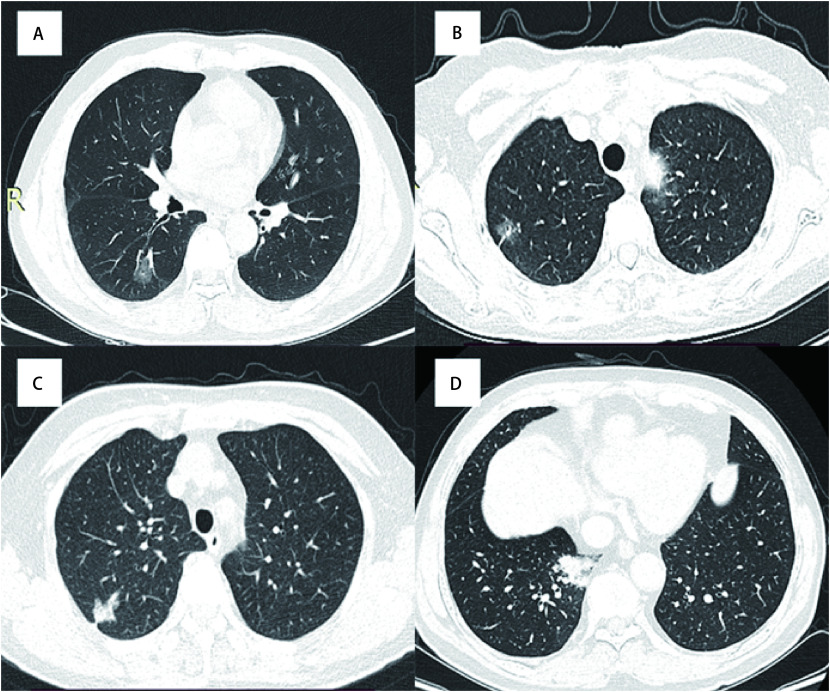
典型的CT征象。A：*EGFR*、*ALK*基因野生型腺癌，以磨玻璃成分为主；B：*EGFR*基因外显子21突变型腺癌，存在胸膜牵拉征及较多磨玻璃成分；C：*EGFR*基因外显子19突变型腺癌，存在分叶征及较多实性成分；D：*ALK*基因突变型腺癌，以实性肿块为主。 Typical CT signs. A: *EGFR* and *ALK* gene wild-type adenocarcinoma, mainly composed of ground glass; B: Exon 21 mutant adenocarcinoma had pleural traction sign and more ground glass components; C: Exon 19 mutant adenocarcinoma had lobulation sign and more solid components; D: *ALK* gene mutant adenocarcinoma is mainly solid mass. CT: computed tomography.
